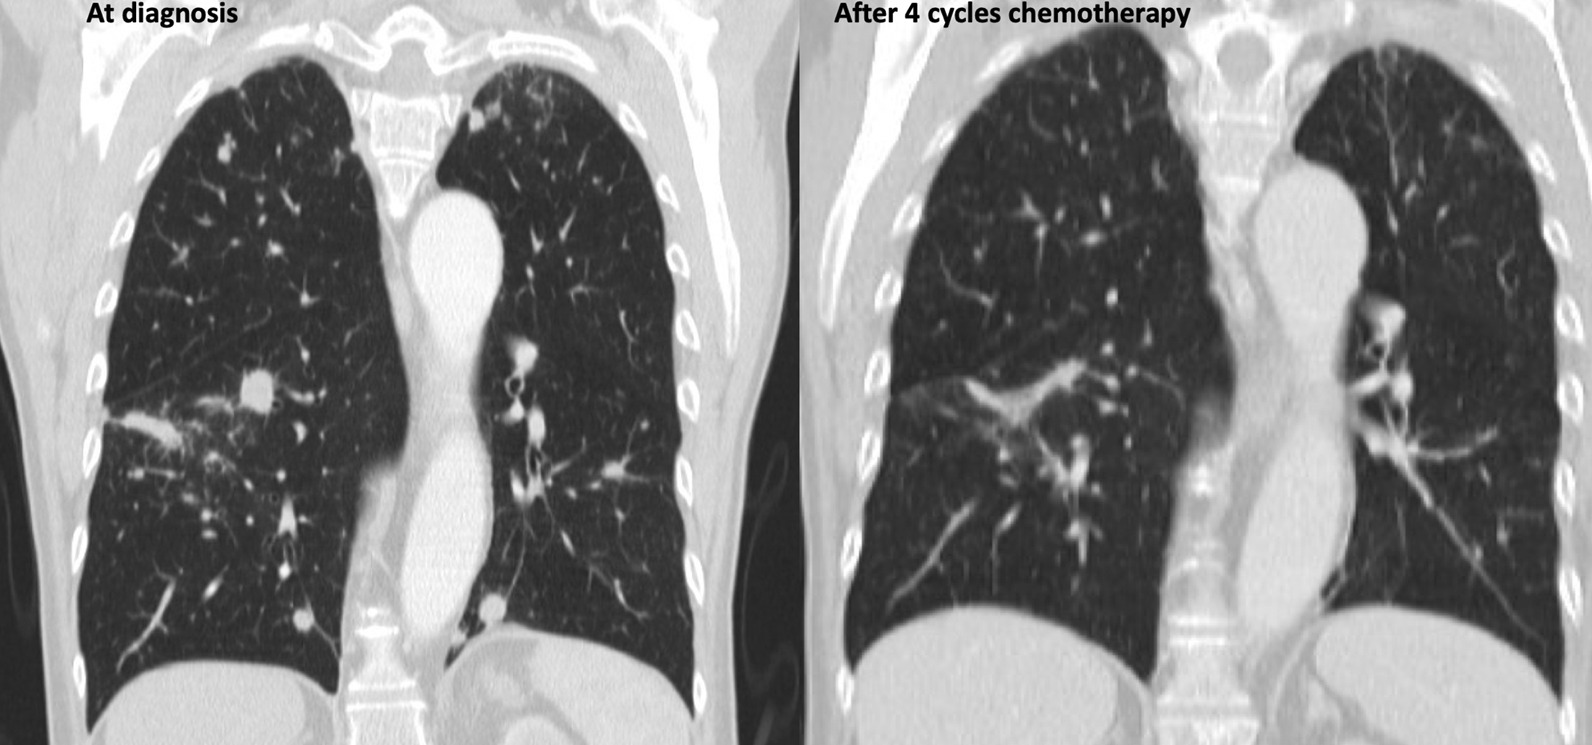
Pretreatment computed tomography scan with the presence of multiple lung metastases from the collecting duct carcinoma of the left kidney at diagnosis (left). Note the remission of the lung lesions after four cycles with gemcitabine/cisplatin chemotherapy (right)
